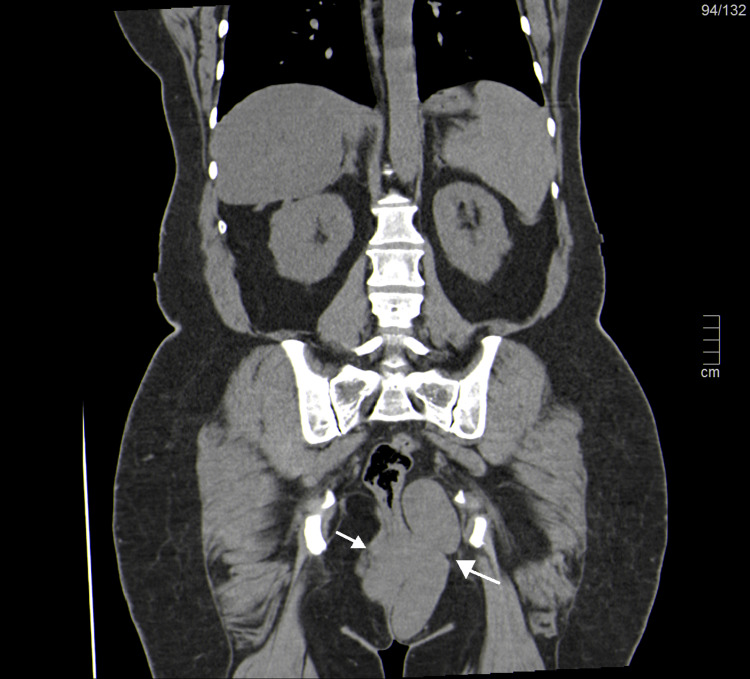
Computed tomography of the coronal reformated plane showing pelvic and vulvar masses (arrows)
